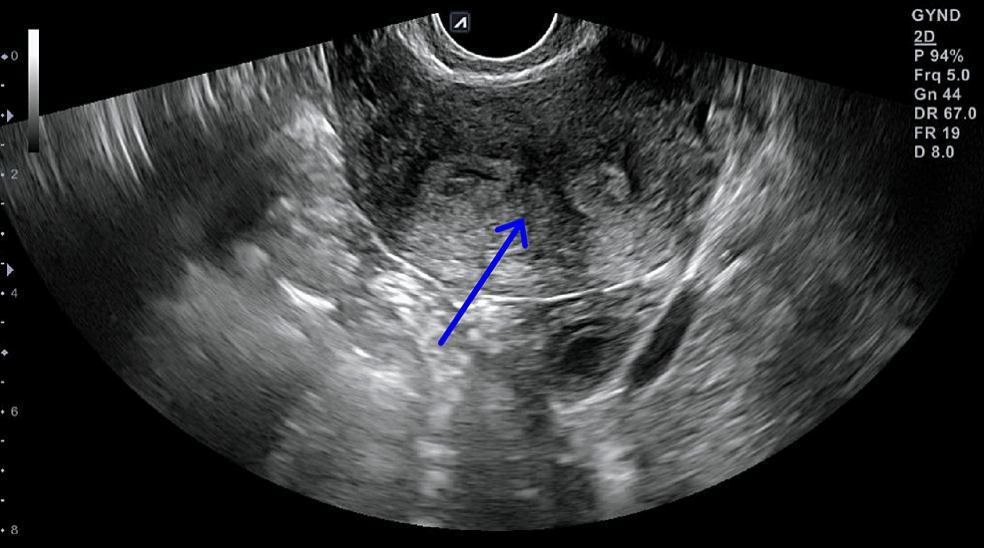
Two-dimensional ultrasound indicates uterine septum.
